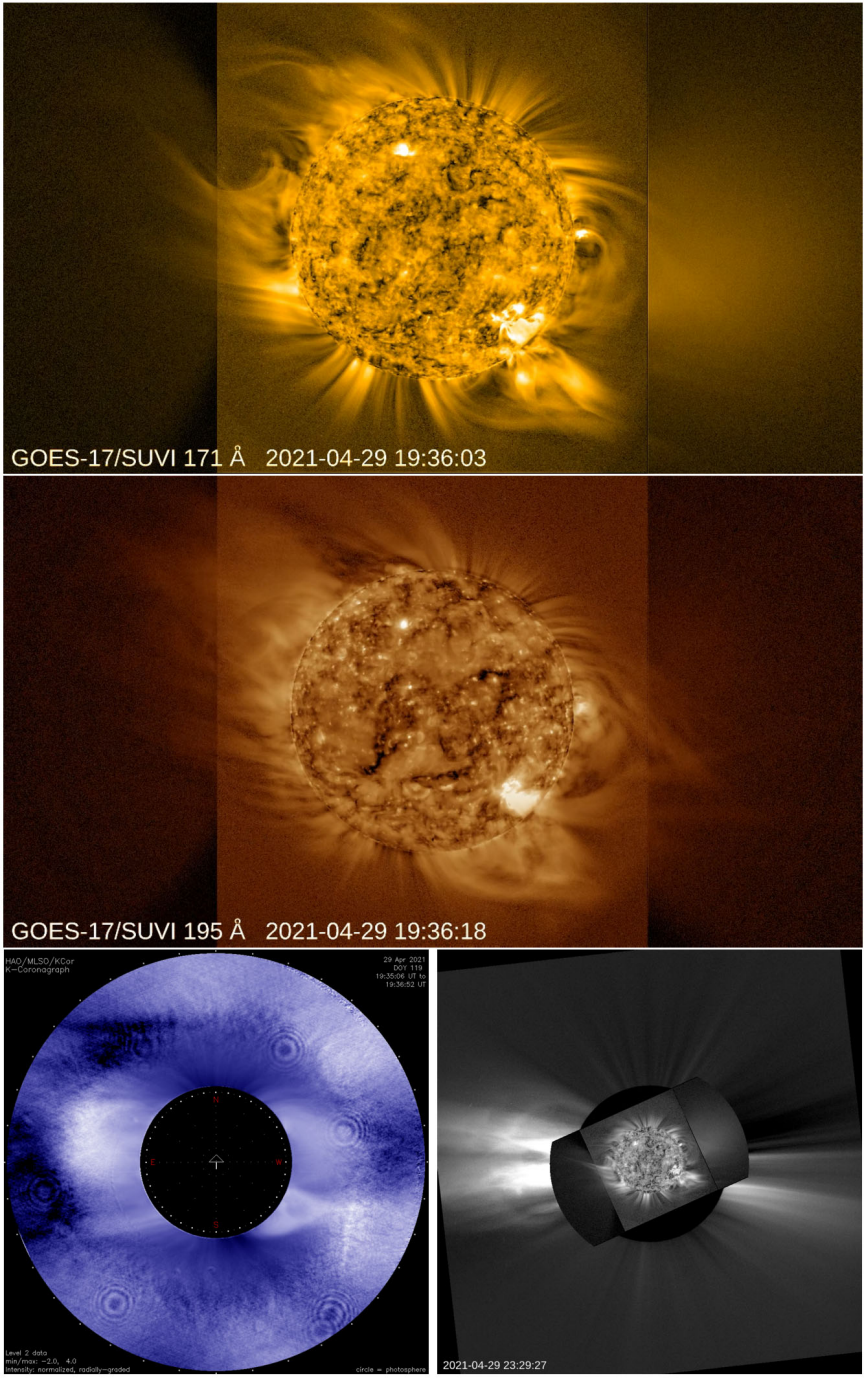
Different views of the middle corona, observed on 29 April 2021, in EUV from SUVI (*top* in 17.1 nm, and *middle panel* in 19.5 nm) and visible light from K-Cor (*bottom left*) and LASCO (*bottom right*; with SUVI superimposed). Images are in camera coordinates and not necessarily co-aligned, although solar North is generally upwards in each frame.
